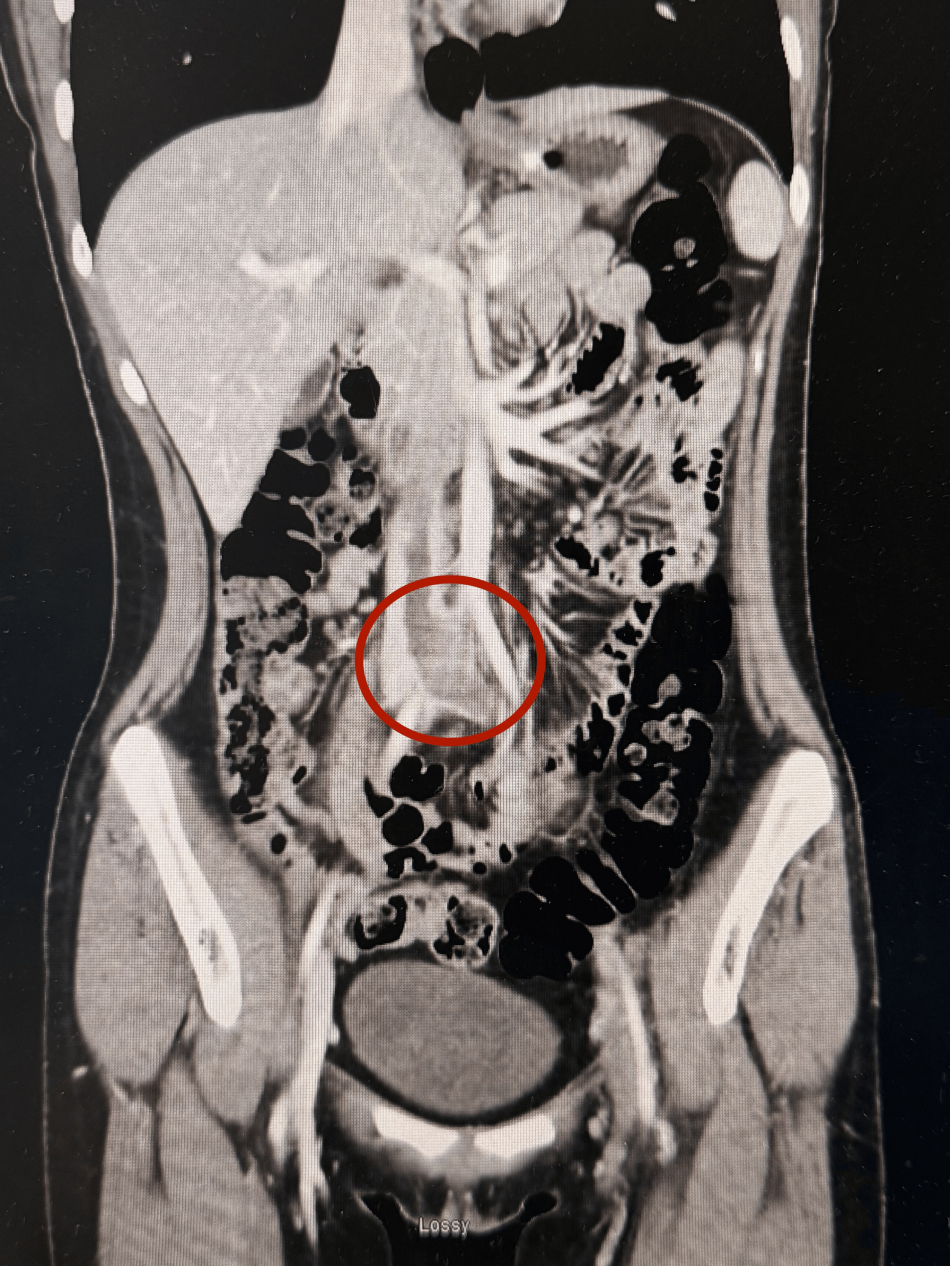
CT angiogram of the abdomen and pelvis (coronal view) Coronal CT angiogram of the abdomen and pelvis demonstrating compression of the left common iliac vein by the overlying right common iliac artery. The red circle highlights the site of thrombosis consistent with May-Thurner syndrome.
